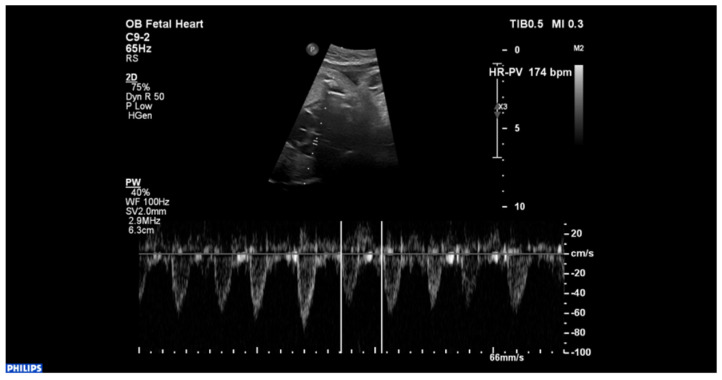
Fetal echocardiogram displaying couplets of premature ventricular beats.
